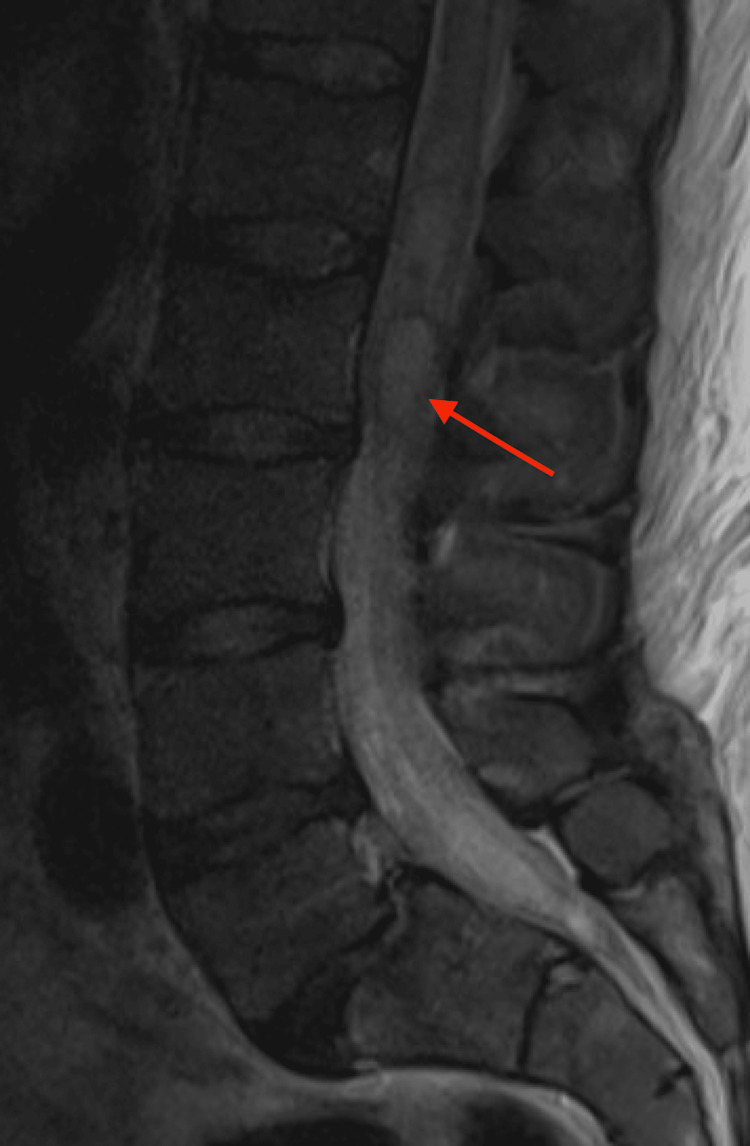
Sagittal lumbar spine MRI T2 image showing multilobulated, hyperintense mass associated at the level of L1-L3 measuring approximately 1.1 cm AP by 0.9 cm by 6.2 cm
